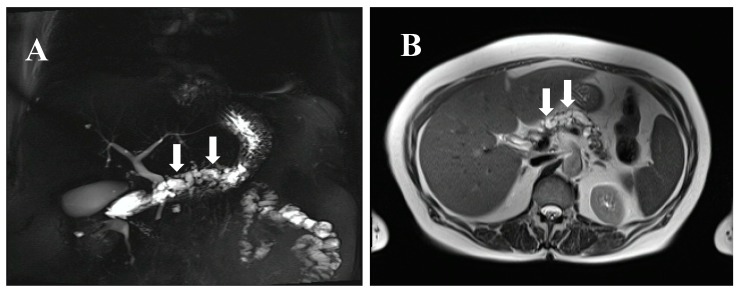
MRCP (magnetic resonance cholangiopancreatography) (A) and MRI (magnetic resonance imaging) (B) of a 77-year-old female individual at risk (IAR) with a cystic dilated Wirsung duct, a potentially main-duct intra-ductal papillary mucinous neoplasia (IPMN) from a familial pancreatic cancer (FPC) family with four affected relatives. The IAR underwent total pancreatectomy and pathological examination revealed a main duct IPMN with multifocal moderate and severe dysplasia. Preoperative serum levels of miR-196b, LCN2, and TIMP1 were strongly elevated and dropped to normal levels at postoperative day 10. Arrows indicate the cystic dilated Wirsung duct.
